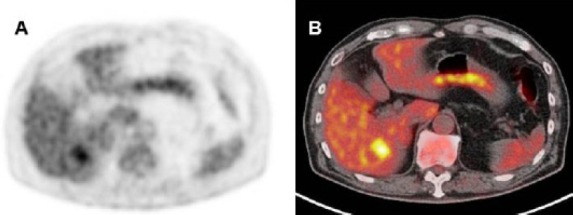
18F-FDG PET image shows an increased uptake in the liver, with a maximum standardized uptake value of 5.04 (A). Fused PET/CT image clearly shows that the accumulation corresponds to a liver mass (B)
